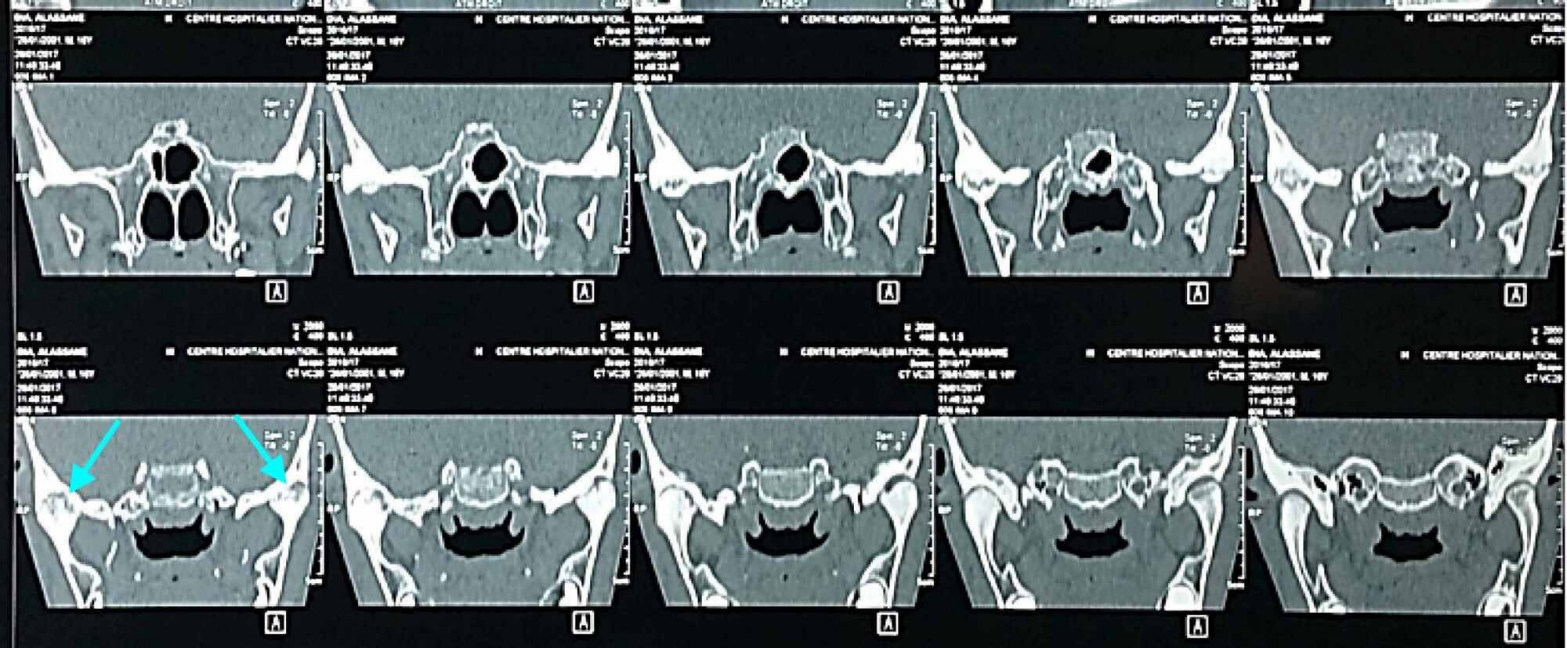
Temporomandibular arthritis Computed tomography showed destructive temporomandibular arthritis with bilateral ankylosis
